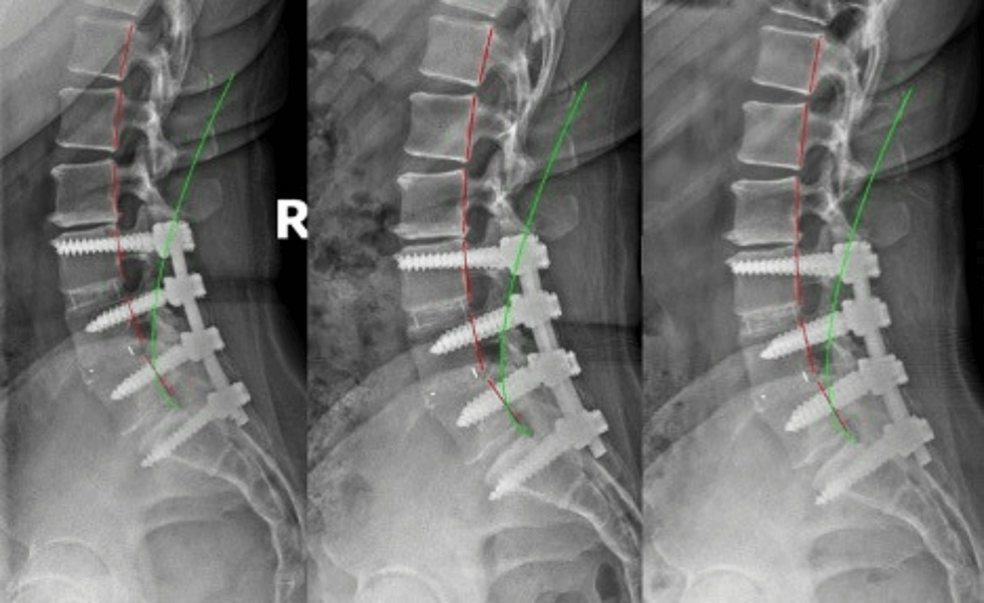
Lateral lumbar X-rays Left: Initial; Middle: First post-treatment; Right: Six-month follow-up. Notice surgical hardware from L3-S1. Green lines indicate an ideal curve; red lines highlight the patient’s spinal alignment. Note the reduction of the horizontal distance between the top of the green line and the posterior-superior corner of the T12 vertebral body after treatment.
